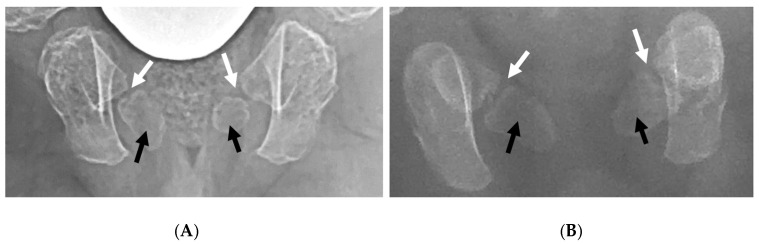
Enlarged anteroposterior pelvic radiographs of 18-month-old (A) and 36-month-old (B) patients with SEDC demonstrating multiple ossification centers as distinct bony parts (black arrows) separated by a radiolucent strip (white arrows).
